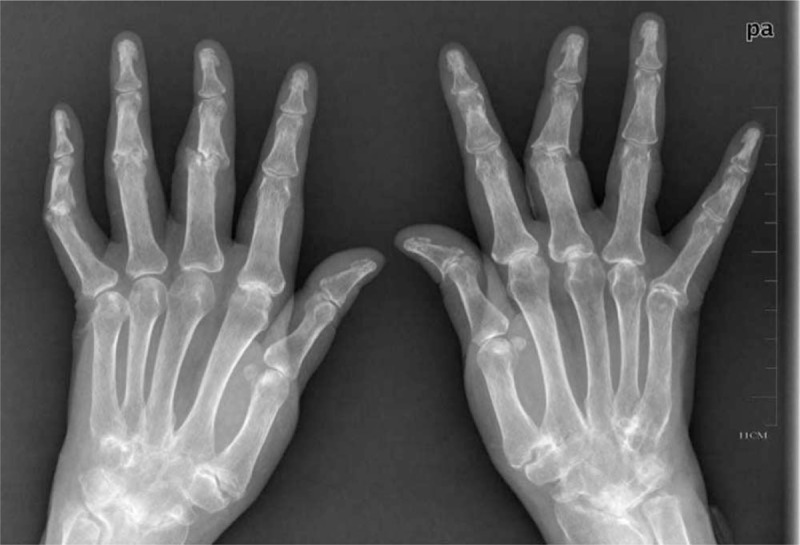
Radiologic erosions. Both hand x-ray images demonstrated advanced arthritis combined with body ankylosis.
